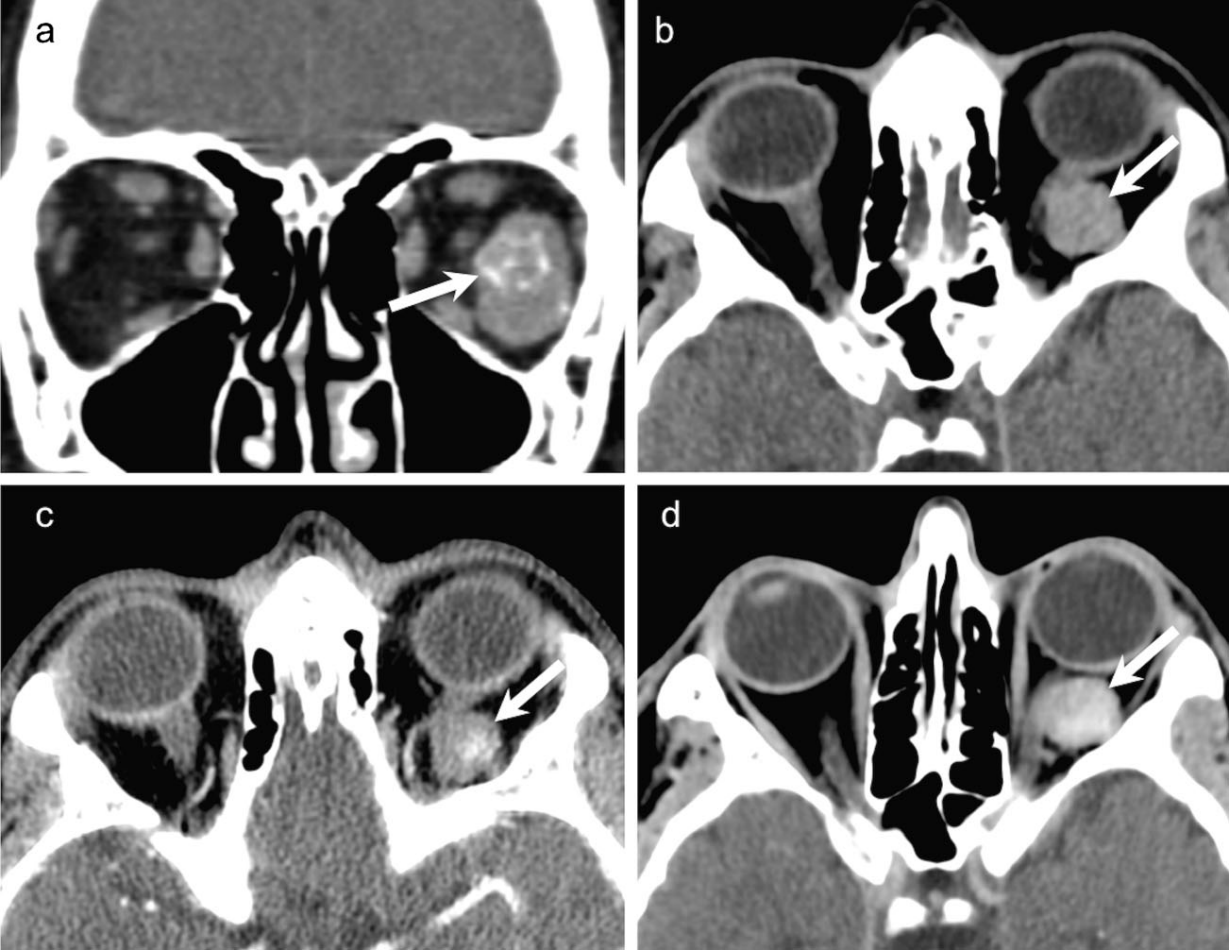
Orbital hemangioma as seen on CT in two different patients. a. Coronal NECT in a 30-year-old male patient shows a well-circumscribed intraconal mass (arrow) with calcified phleboliths. b-d. 50-year-old male patient with an incidentally diagnosed left orbital cavernous hemangioma on an angio-CT performed for stroke. b. NCECT shows a nonspecific intraconal lesion (arrow) without phleboliths. Arterial phase (c) demonstrates initial patchy enhancement (arrow) followed by progressive filling of the lesion in the venous phase (d)
